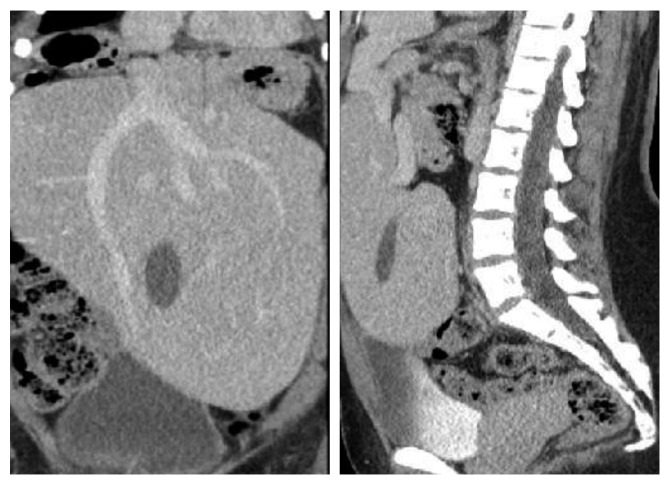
Coronal and sagittal computed tomography pictures showing the pelvic location of the liver and the intrahepatic location of the gallbladder.
